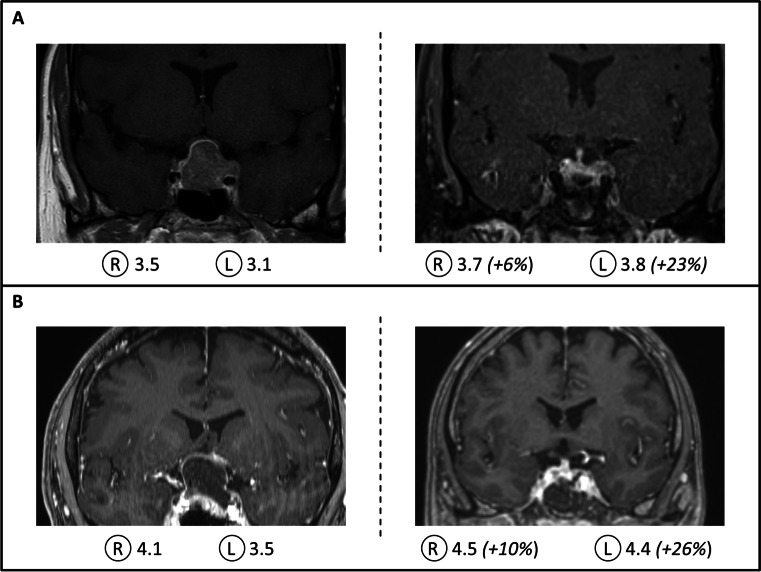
Exemplary results of patients with compression of the optic chiasm. Patient A experienced impaired vision due to a solid pituitary adenoma with compression of the optic chiasm on magnetic resonance imaging (MRI) studies (left). The neurological pupil index (NPi) values were < 4.0 (R, right; L, left) in both pupils and improved considerably after surgical tumor resection (right). Intraoperative MRI demonstrated sufficient decompression of the optic chiasm. Patient B presented with a cystic pituitary adenoma with compression of the optic chiasm (left). Corresponding to the improved visual acuity after surgery, the NPi values improved, and intraoperative MRI demonstrated sufficient decompression of the optic chiasm (right)
